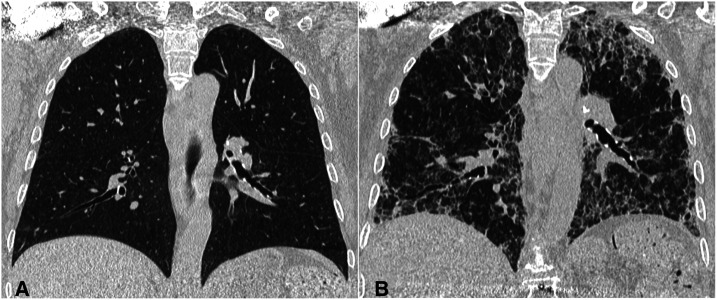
Chest computed tomography coronal reconstructions: (A) executed in 2019, showing no abnormalities; (B) executed in 2020, after COVID-19 infection. Diffuse lung architectural distortion is visible.
